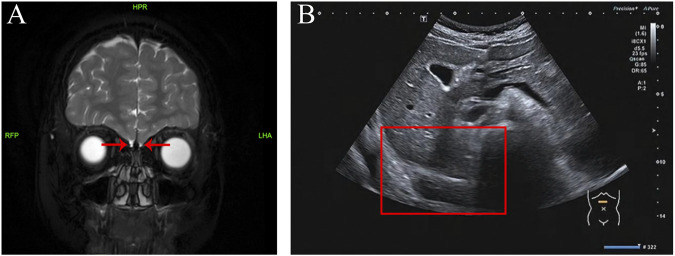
MRI and ultrasound images of the affected male patient (IV14). (A) Coronal thin-section T2-weighted images demonstrated no distinct nerve signal within the olfactory grooves bilaterally (indicated by red arrows). (B) Abdominal ultrasonography revealed absence of the right kidney in the right renal fossa, suggestive of right renal agenesis (indicated by red box).
